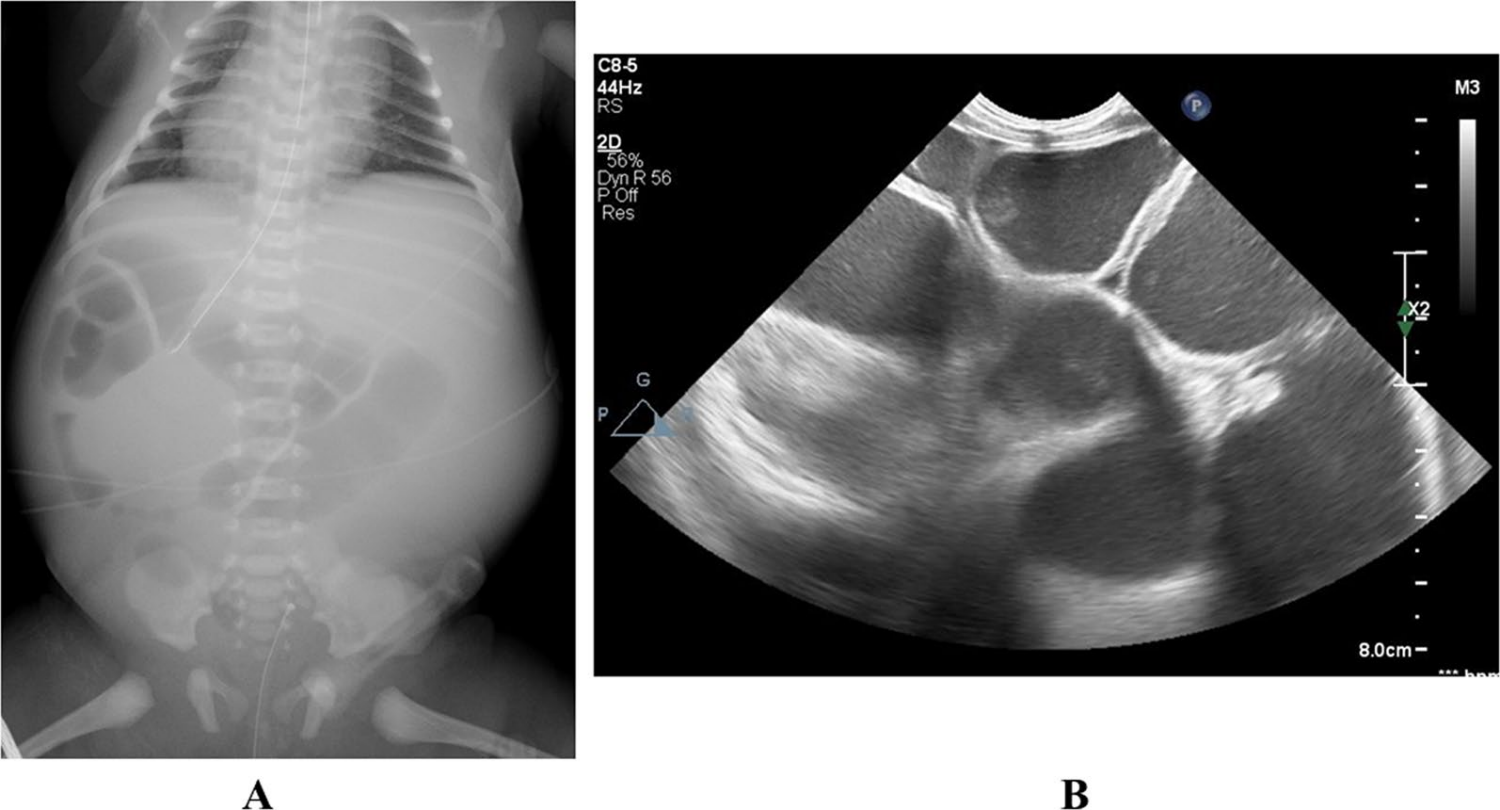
Preoperative imaging studies. A X-ray film revealed dextrocardia, opacity of the liver on the left side, and shifting of the nasogastric tube to the right side. B Abdominal ultrasonogram showed remarkable dilatation of the intestine
